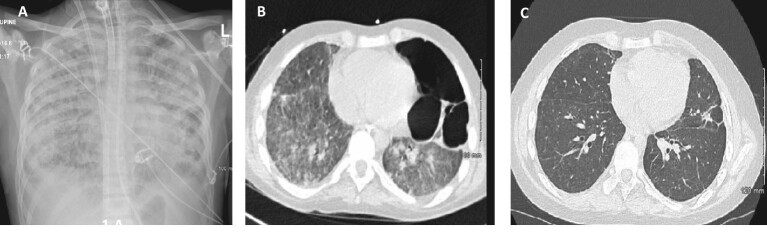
(A) Patient 2 after the first month: chest X-ray: bilateral interstitial infiltrates; (B) Patient 2 after 2 months: chest CT scan: diffuse ground-glass opacity of the lungs, residual left apical pneumothorax, loculated left lateral/basal hydropneumothorax; (C) Patient 2 after 6 months: chest CT scan: significantly improved diffuse ground glass opacification with subtle residual diffuse increased lung density, multiple diffuse bilateral predominantly sub-solid ground glass nodules, residual areas of scarring/atelectasis and traction bronchiectasis.
